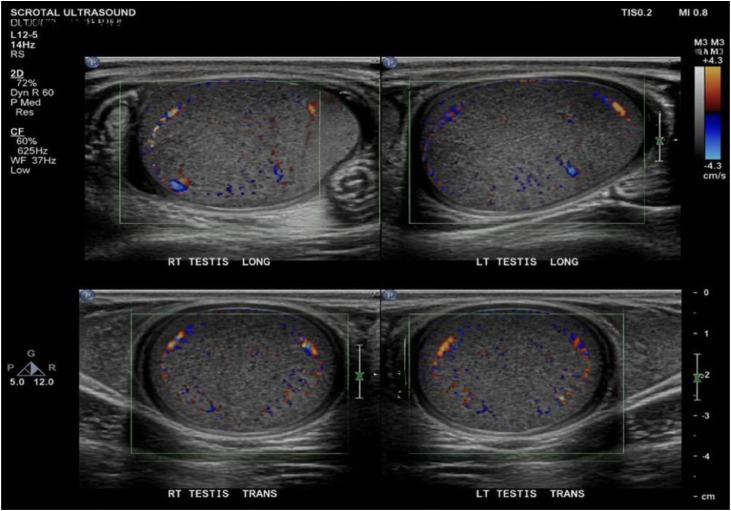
Scrotal ultrasound findings.
